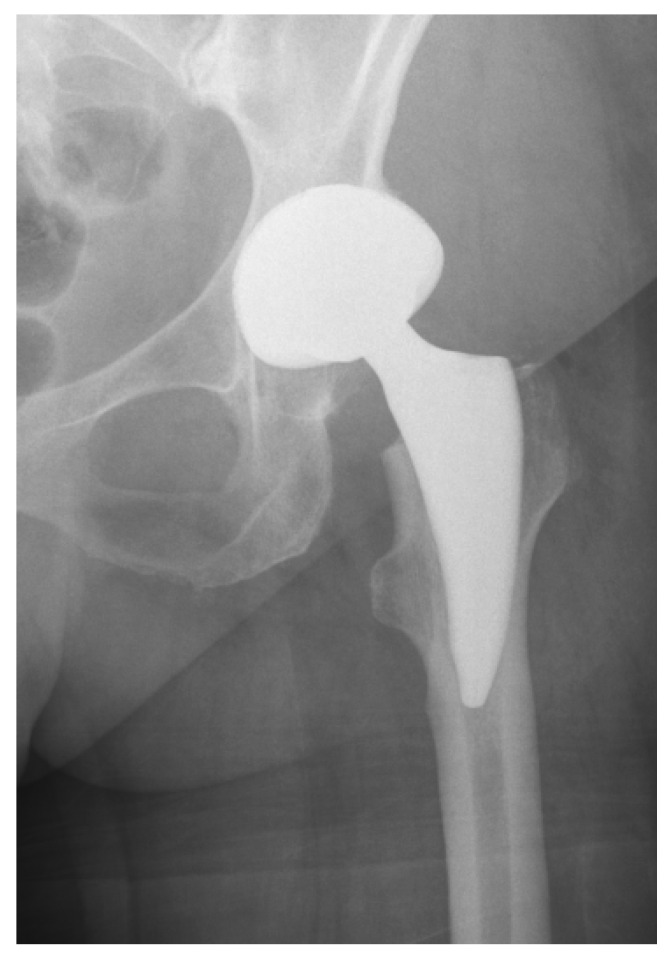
Anteroposterior radiograph of the same patient on the first day after cementless total hip arthroplasty with the Delta TT cup and Minima stem size 1, placed in a neutral position (alignment).
